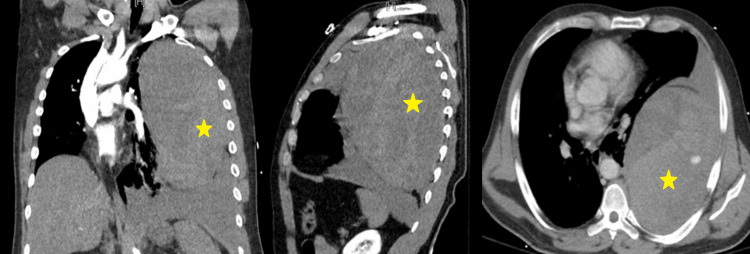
CT imaging demonstrating a large left hemothorax and retropleural hematoma, accompanied by right mediastinal shift, as observed in the coronal, sagittal, and axial planes, respectively.
